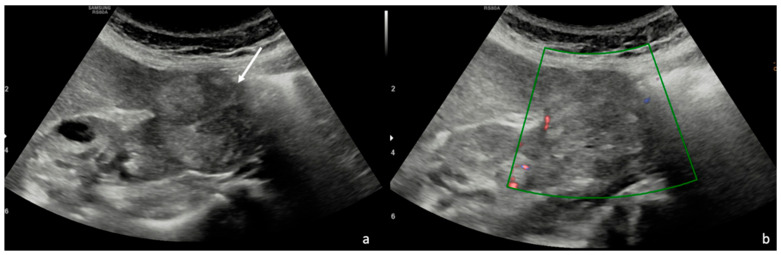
(a) B-mode ultrasound shows an exophytic solid renal lesion (white arrow). (b) Color–Doppler shows no intralesional significant vascularization.
